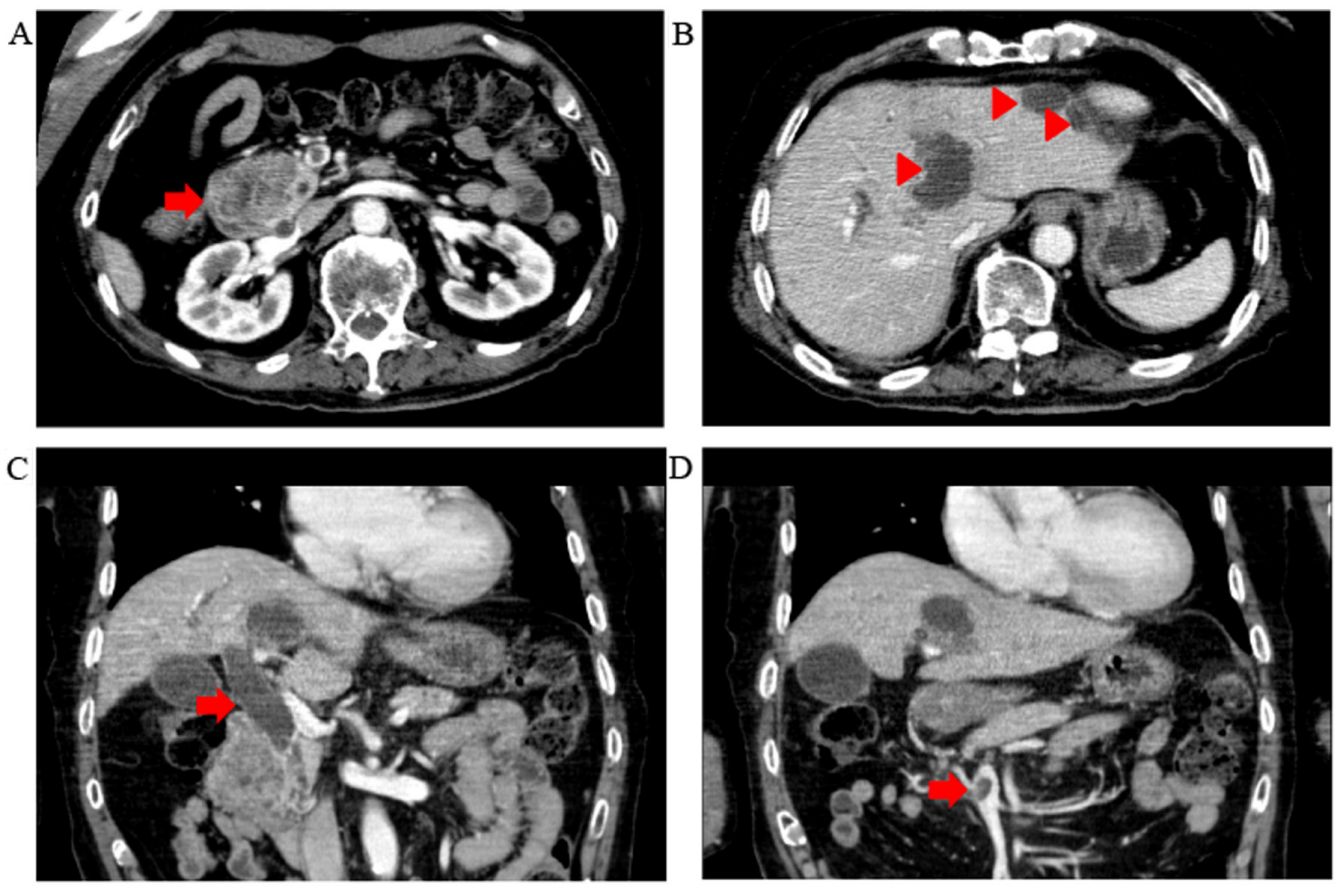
Abdominal contrast-enhanced CT at the patient's first visit. (A) A polycystic tumor was identified in the pancreatic head (arrow). (B) Polycystic low-density areas in the liver (arrowheads). (C) The pancreatic tumor obstructed the common bile duct, and the proximal part of the bile duct was dilated to 20 mm (arrow). (D) A 10-mm area with lack of enhancement was identified in the superior mesenteric vein (arrow).
